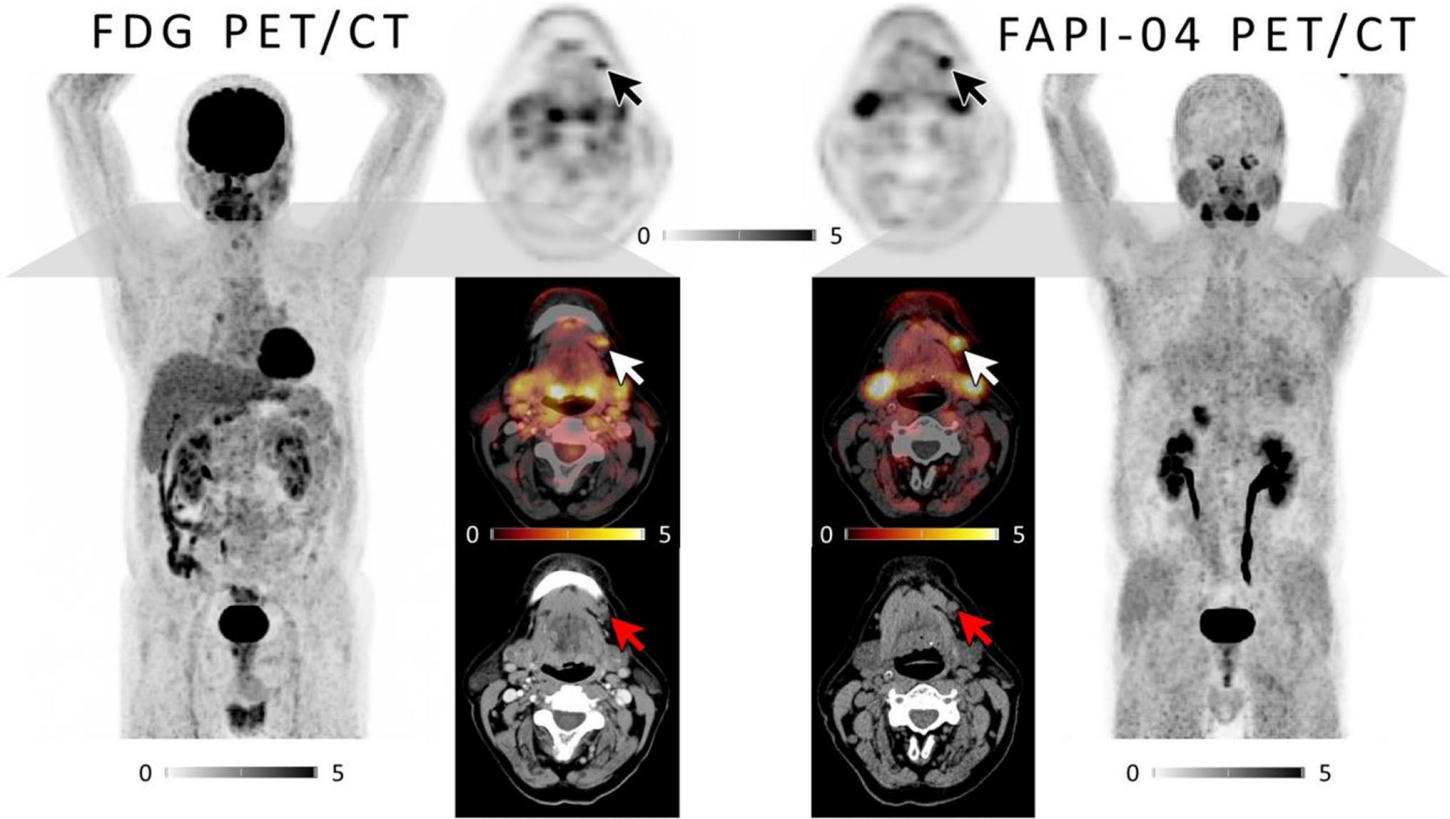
Comparison of [^18^F]FDG and FAP-directed [^68^ Ga]FAPI-04 PET/CT in a patient with newly diagnosed, treatment-naïve oral squamous cell carcinoma and a cervical lymph node metastasis. Maximum Intensity Projections (MIP, outer columns) as well as axial PET (top), fused PET/CT (middle) and CT (bottom) slices of [^18^F]FDG (left) and [^68^ Ga]FAPI 04 (right) PET scans in a patient with newly diagnosed, treatment-naïve squamous cell carcinoma of the alveolar process of the mandible (patient #1). Whereas both tracers detect the regional lymph node metastasis in cervical level Ib, FAP-directed imaging offers higher tracer uptake. Of note, neither tonsils nor other lymph nodes are [^68^ Ga]FAPI-04 PET-positive in this example
